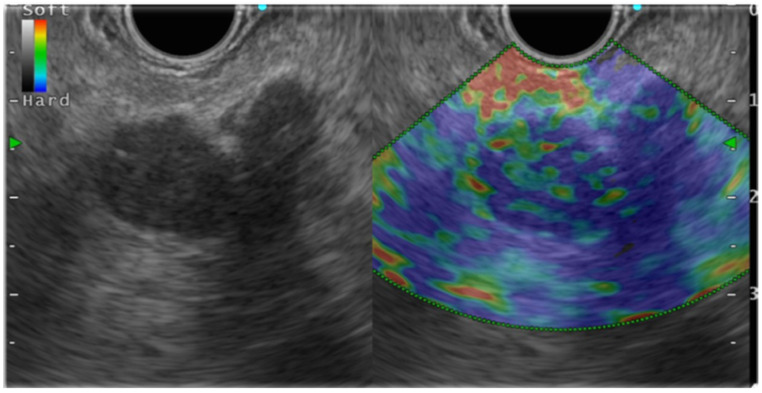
Endosonography(EUS) elastography in pancreatic ductal carcinoma.
